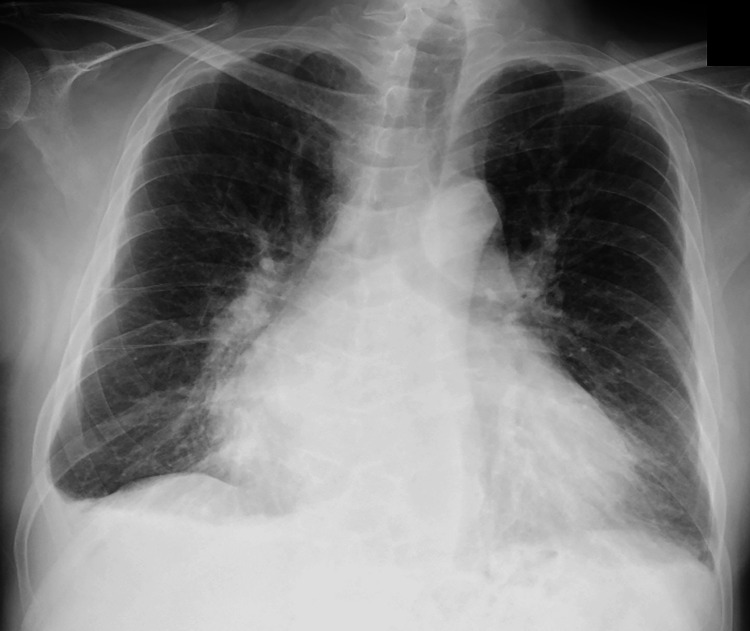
Chest X-ray on admission Chest radiography showed a cardiothoracic ratio of 70% and bilateral pleural effusion.
